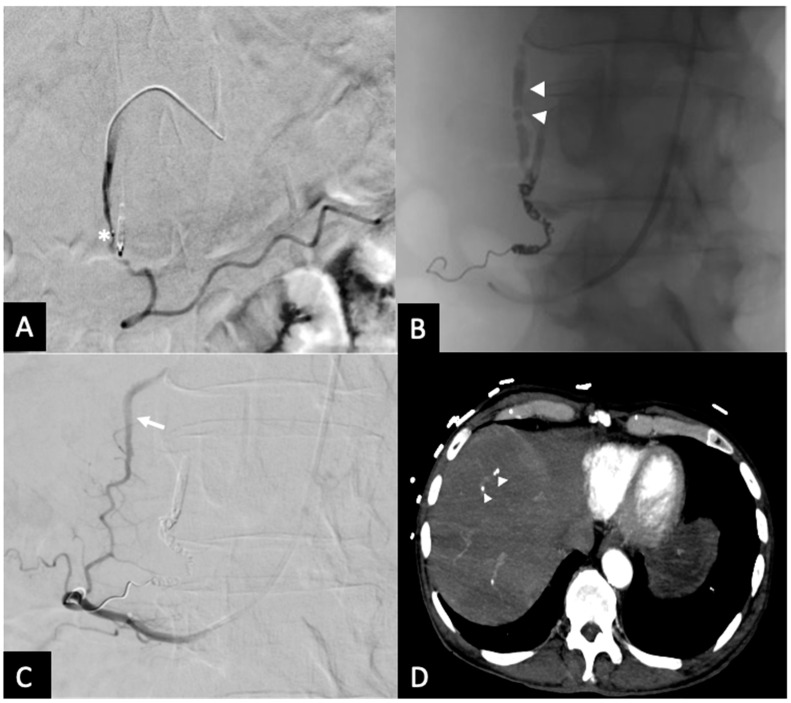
Rebleed example of unembolized RGEA—(A) Pre-embolization radiograph demonstrating the GDA and endoluminal clip placed by during endoscopy (*). (B) A single coil was placed in distal GDA, with Ta-OCE extending to proximal GDA (arrowheads). (C) Repeat angiography from the SMA approach upon the patient’s continued UGI bleeding demonstrated patent collateral flow from the posterior pancreaticoduodenal arcade (arrow). (D) Follow-up CT obtained between the 2 embolization procedures demonstrating separation of Ta-OCE (arrowhead) and embolization of branch hepatic arteries. Image obtained from patient’s chart. Source: NK.
